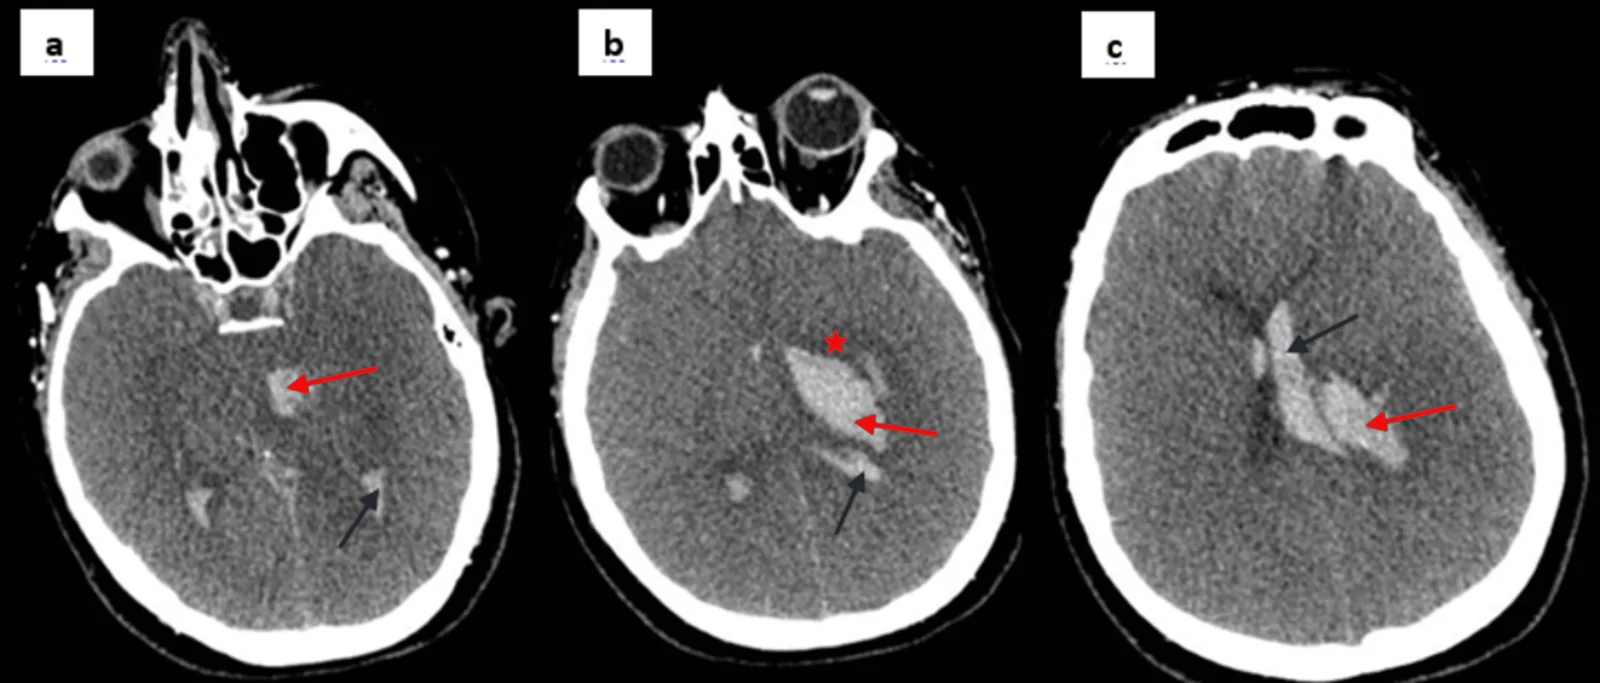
CT scan demonstrating a deep capsulolenticular intraparenchymal hemorrhage (red arrows in a, b, and c) extending into the brainstem, associated with extensive intraventricular hemorrhage (black arrows in a, b, and c), diffuse cerebral edema (red asterisk * in b), and subfalcine herniation.
